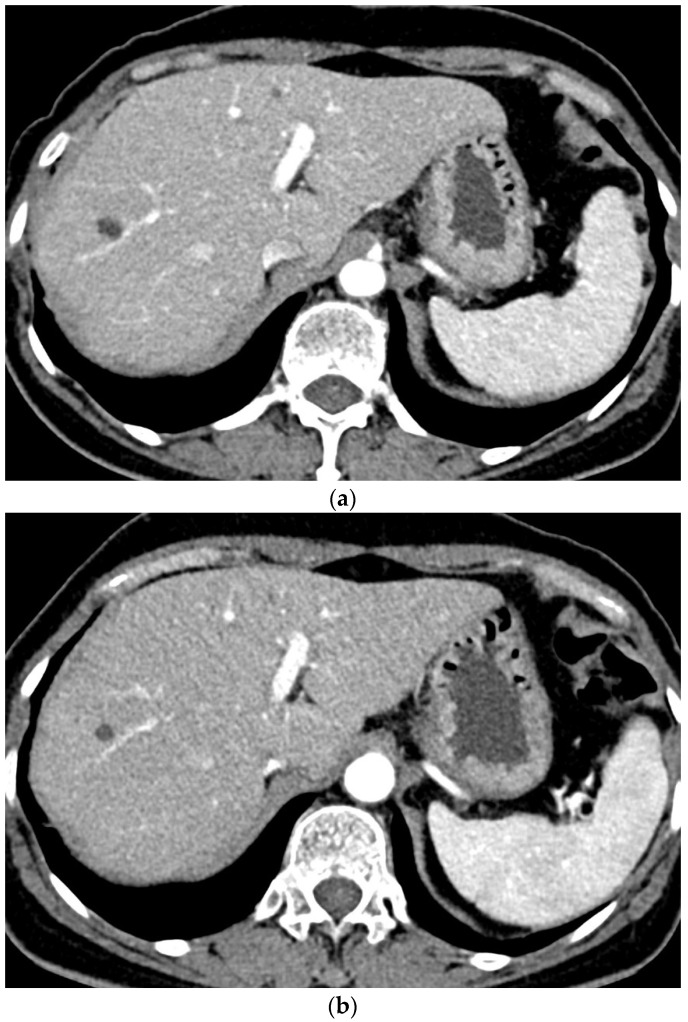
A 64-year-old female patient who underwent CT examinations using the same machine (machine B). An approximately 0.7-cm cyst is noted in liver segment 8 on both CT examinations with 240 mgI/mL contrast material (a) and 320 mgI/mL contrast material (b).
